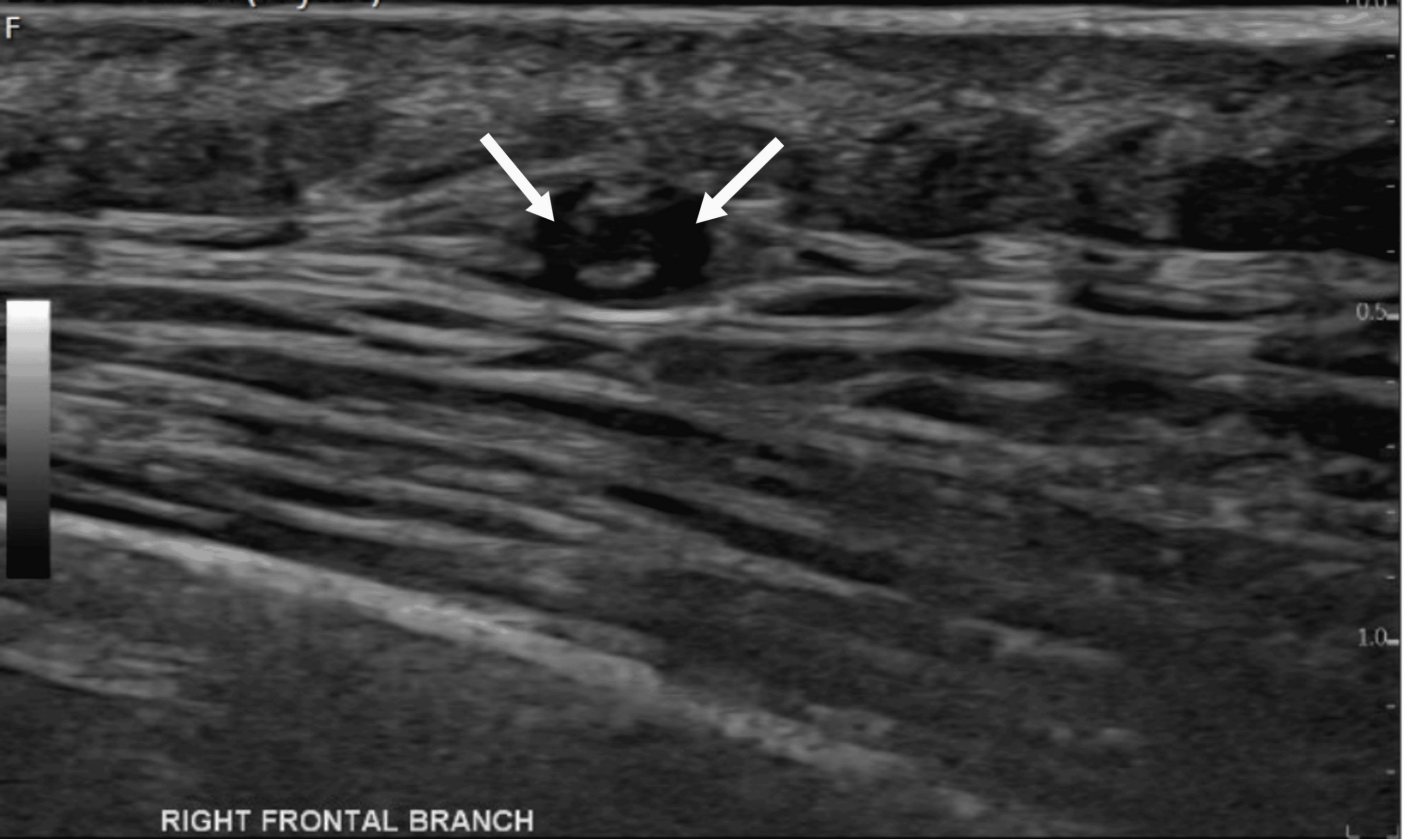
The right frontal branch US Doppler Positive compression sign, the hypoechoic area persists (arrow) during the compression maneuver of the vessel lumen US: ultrasound
